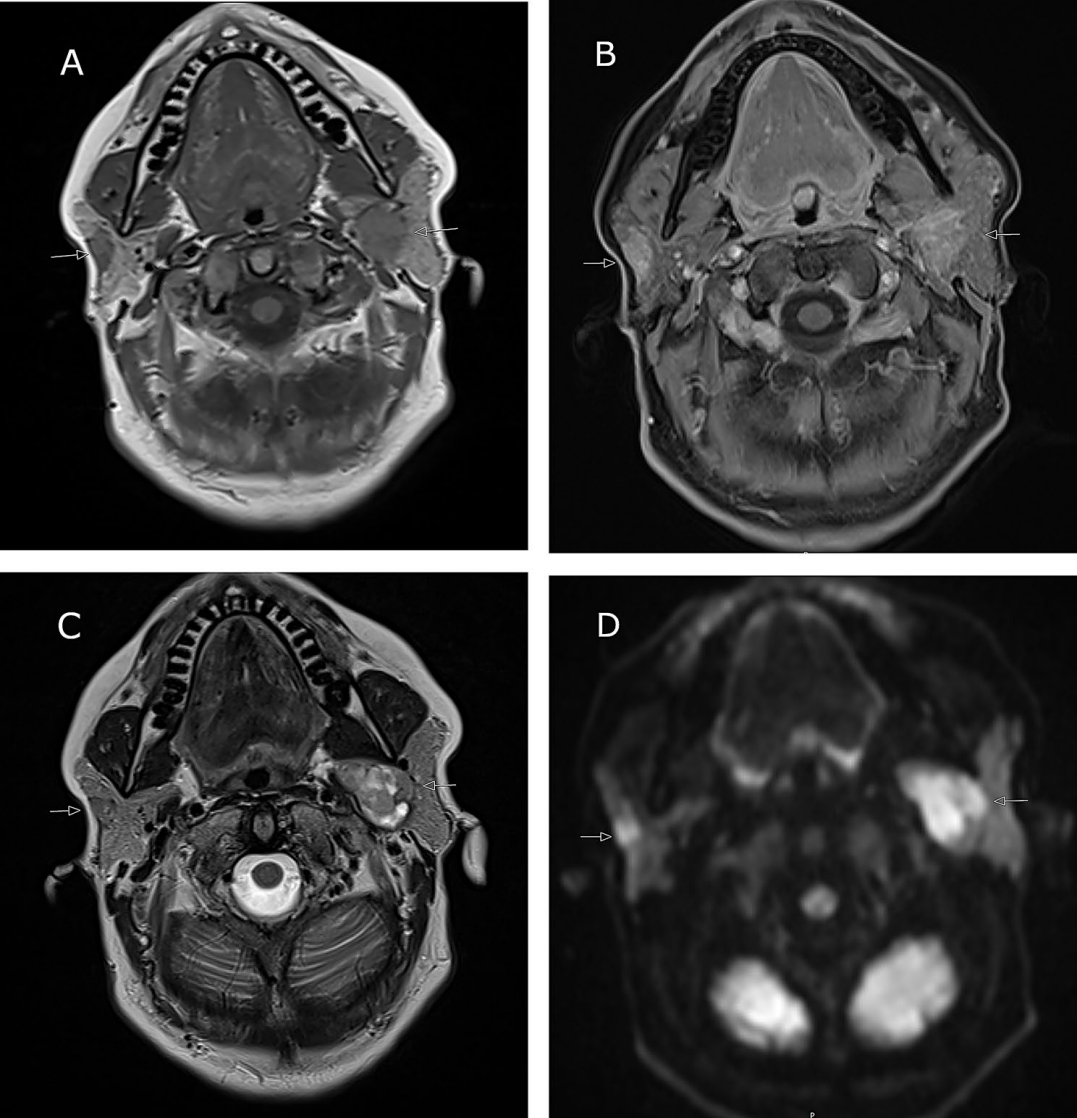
Patient 3, MR image of bilateral salivary gland tumors in a 51-year old female. A-D Bilateral intraparotid lesions with irregular borders and similar textures on the left side in the deep lobe and on the right side in the superficial lobe (arrows). A) Low signal on T1W, B) heterogeneous contrast enhancement on fat-suppressed TIW, C) mixed signal on T2W and D) restricted diffusion on DWI; the ADC was bilaterally approximately 1,1 × 10^− 3^ mm^2^/s (not shown). E) MR examination 15 months after surgery reveals a small subcutaneous recurrence (arrow) on the right side (fat-suppressed T1W with contrast enhancement)
